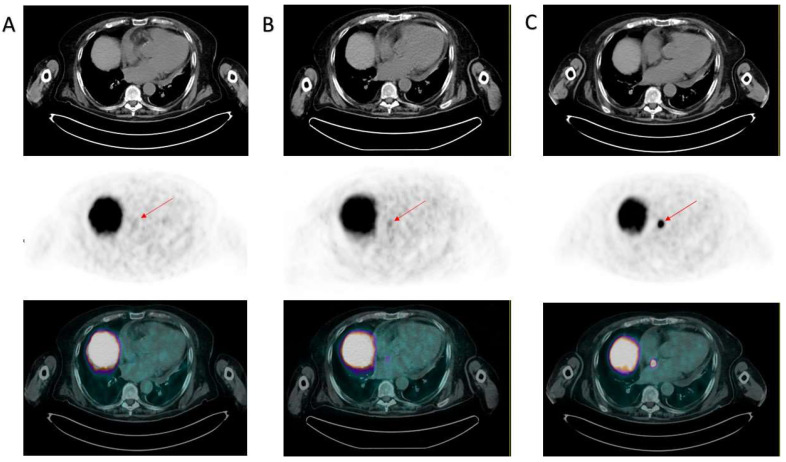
Evolution of the interatrial septum lesion. PSMA PET/CTs from January 2022 (A), June 2022 (B), and October 2022 (C) are displayed. All panels from the upper to the lower images represent axial CT, PET, and hybrid PET/CT obtained at the same slice displayed in Figure 1. The red arrows indicate the location of the interatrial metastasis, which was absent in January 2022 (A), was displayed as a site of minimum (though not significant) tracer uptake in June 2022 (B), and was clearly evident in October 2022, with a maximum SUV equal to 20 (C).
